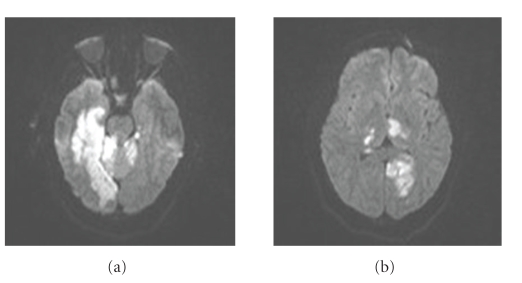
Diffusion weighted MRI demonstrates several confluent areas of restricted diffusion, consistent with infarction, confined to the bilateral posterior circulation (a,b). This was confirmed on apparent diffusion coefficient maps (not shown). No restricted diffusion was present in the anterior circulation (not shown).
